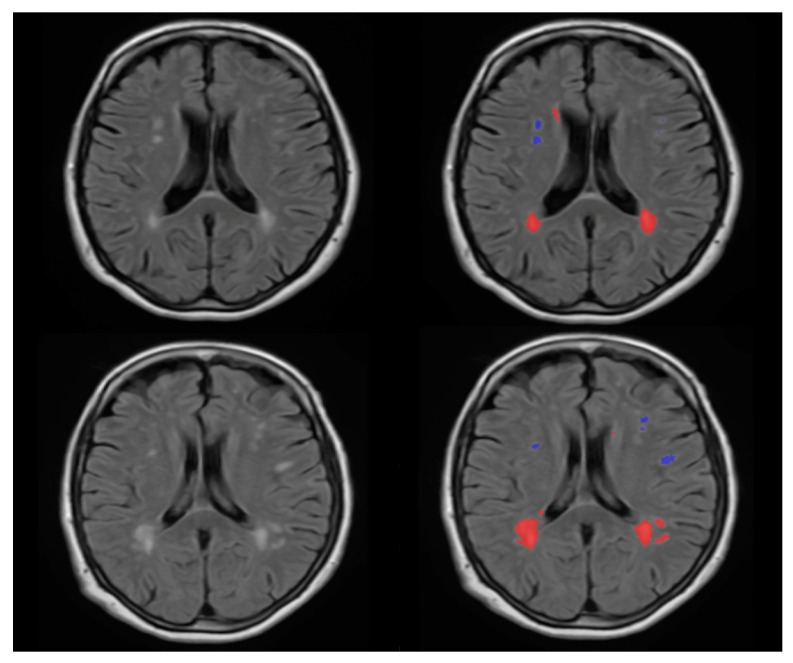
FLAIR MRI imaging of 69 years old female dizygotic twins (left column); the right side column pictures show the segmented version of the FLAIR images. The difference in the anatomy and number of WMH of the cut is visible in this image. According to our analysis, one twin has a total number of 24 WMHs (first row) and the other pair has a total number of 33 WMHs (second row). The red color represents the periventricular WMH and the blue color represents the juxtacortical WMH—image from the Semmelweis University Medical Imaging Centre.
